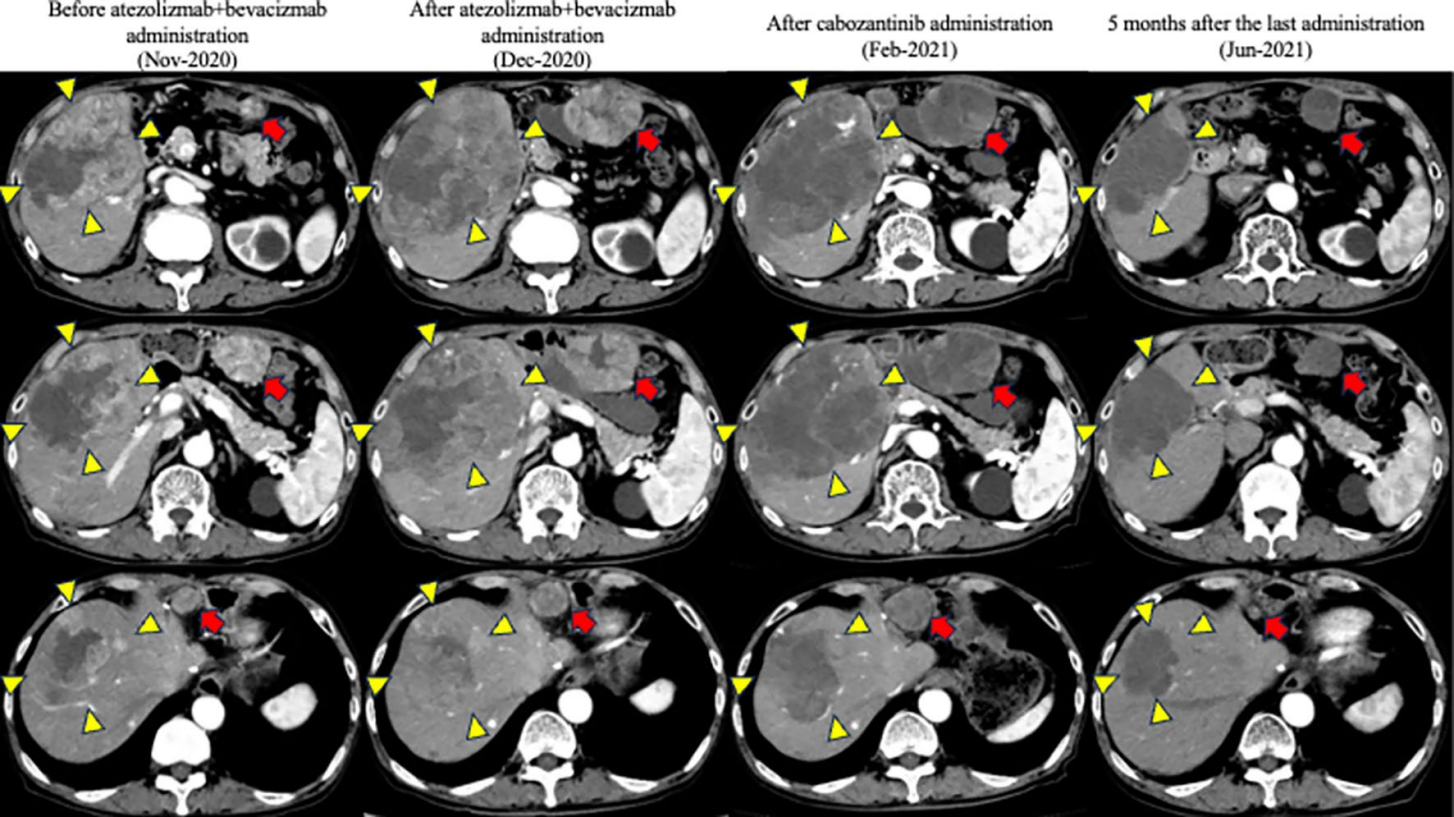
Changes in contrast-enhanced computed tomography image after treatment in our hospital
